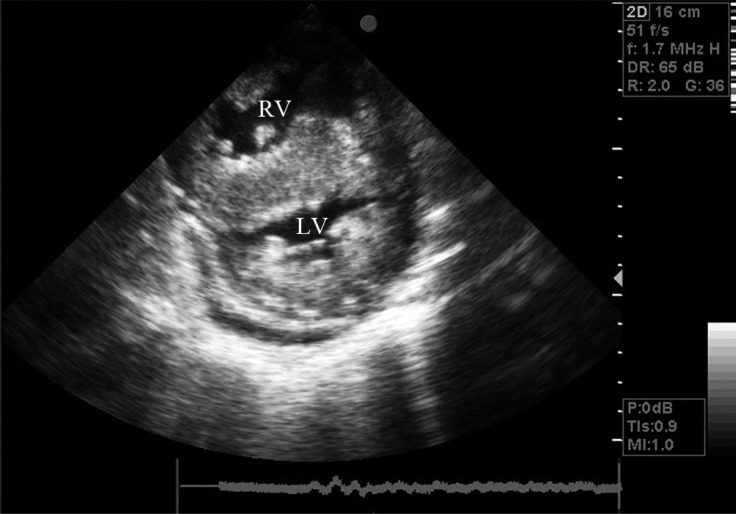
Echocardiography in short-axis view, showing concentric hypertrophic cardiomyopathy.
